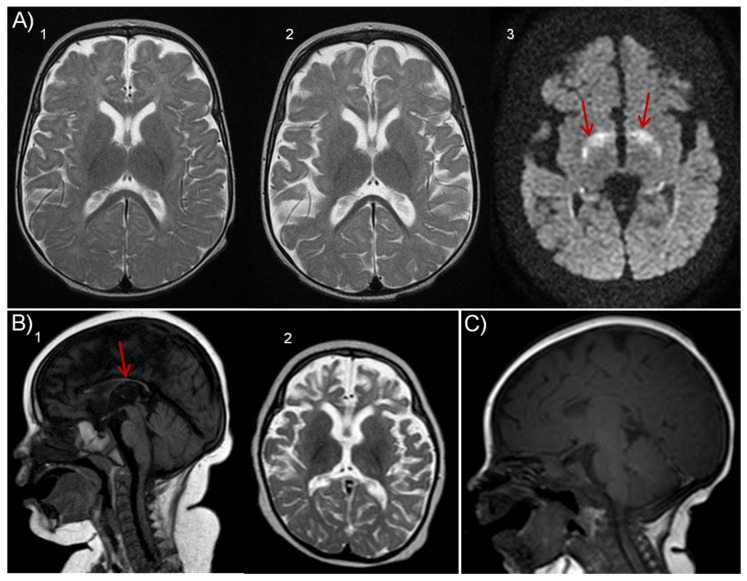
Brain imaging. (A) Patient 1.2: T2 axial MR images show cortical atrophy evolution from the initial MR (1) and after 3 months evolution (2); (3) diffusion-weighted image shows increased paleo-lenticular signal intensity. (B) Patient 1.1: Sagital T1 demonstrate corpus callosum thinning (1) and diffuse cortical and subcortical atrophy in axial T2 (2). (C): Patient 2.1 Sagital T1 image depicts corpus callosum thinning.
